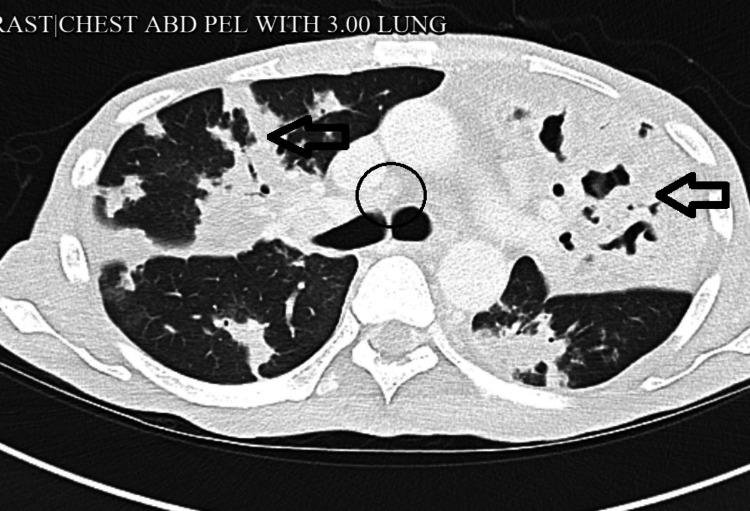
CT scan of the chest showing large bilateral upper-lobe lobulated thick-walled pulmonary cavities compatible with cavitating tuberculosis (black arrows) with lymphadenopathy (black circle) CT, computed tomography
